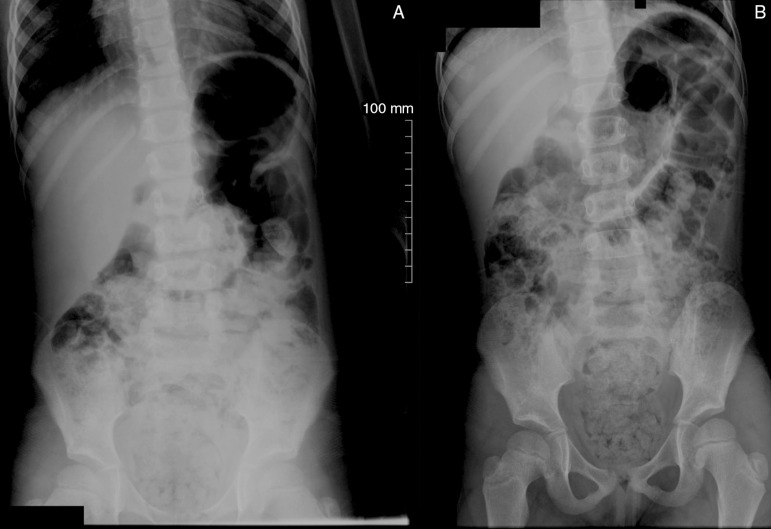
Abdominal X-rays in the standing (A) and supine (B) position, disclosing large
